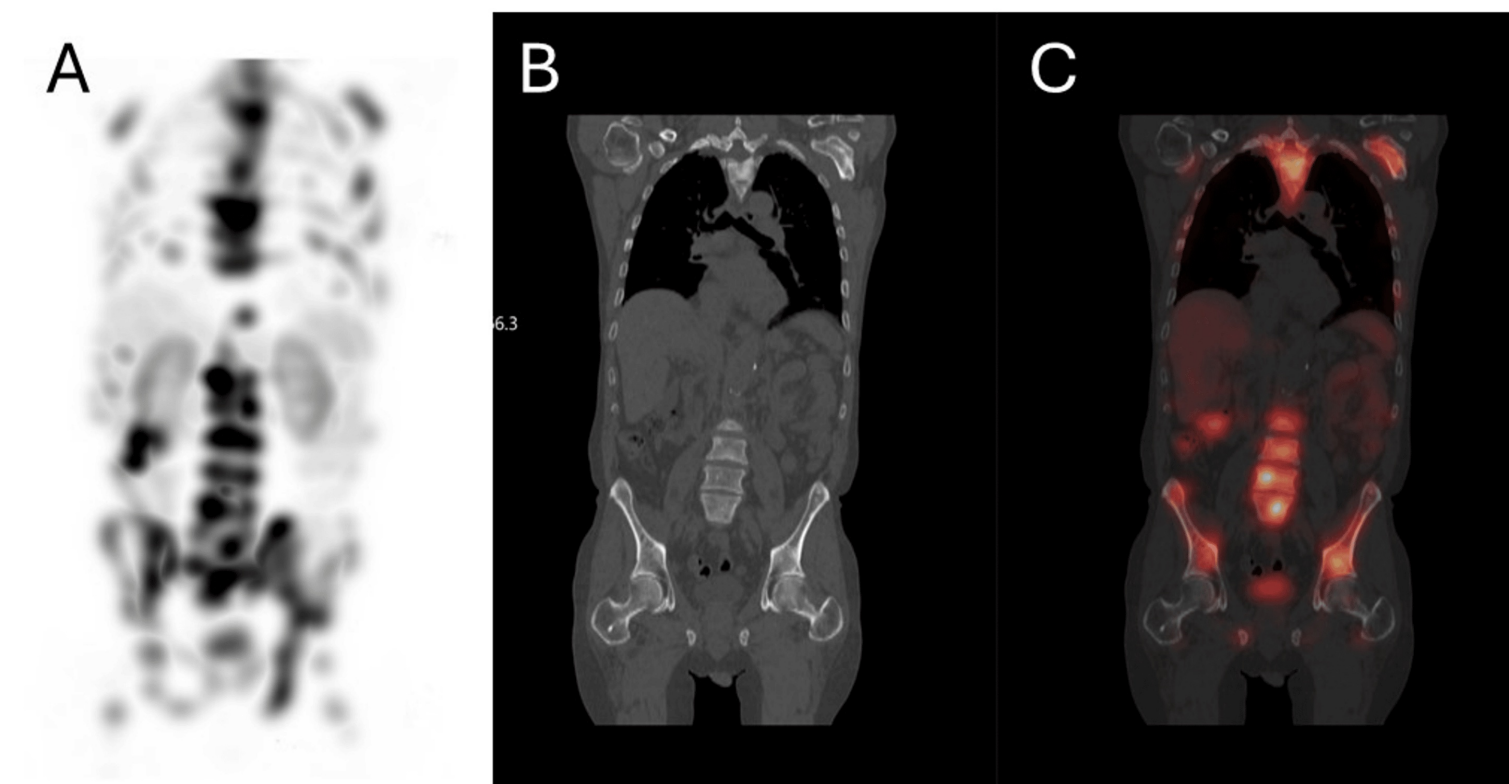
PET after one dose of Lu-177 vipivotide tetraxetan. Post-therapy imaging. A. 3D SPECT image; B. Coronal CT image; C. Fused SPECT/CT image. Single-photon emission tomography/computed tomography (SPECT/CT) imaging was performed two days following the IV administration of 206 mCi of Lu-177 vipivotide tetraxetan, demonstrating radioligand delivery to the osseous lesions, consistent with the radiotracer distribution previously seen on pre-therapy PET/CT imaging. The combination of Ga-68 PSMA-11 PET/CT imaging and Lu-177 vipivotide tetraxetan therapy represents a unique theragnostic approach pairing a diagnostic biomarker with a therapeutic radioligand that shares a molecular target of the cancer tissue.
